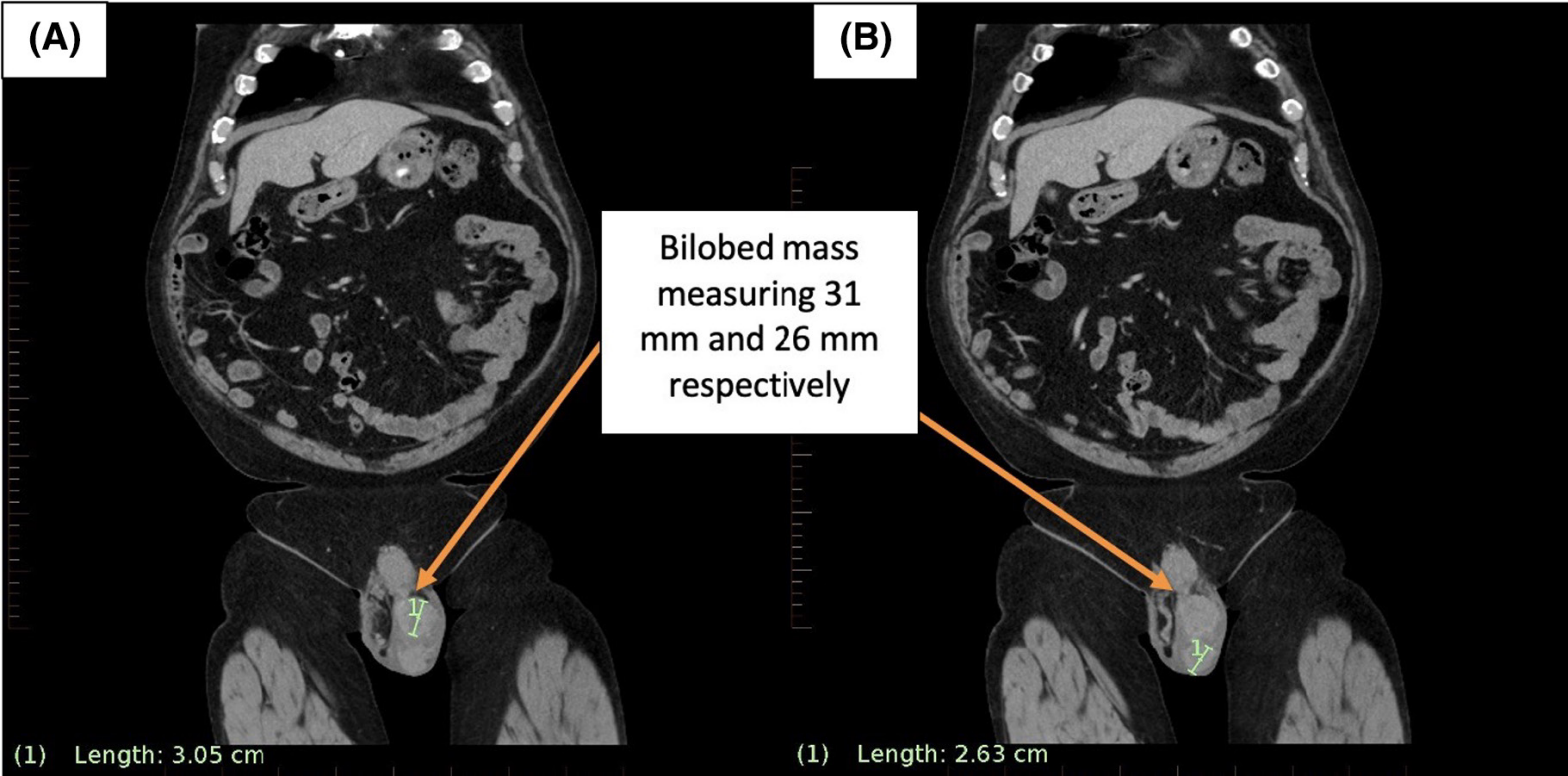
Pelvis CT demonstrating a bilobed mass compressing the left testicle, measuring 31 mm (A) and 26 mm (B) in size respectively, in keeping with ultrasonographic findings.
